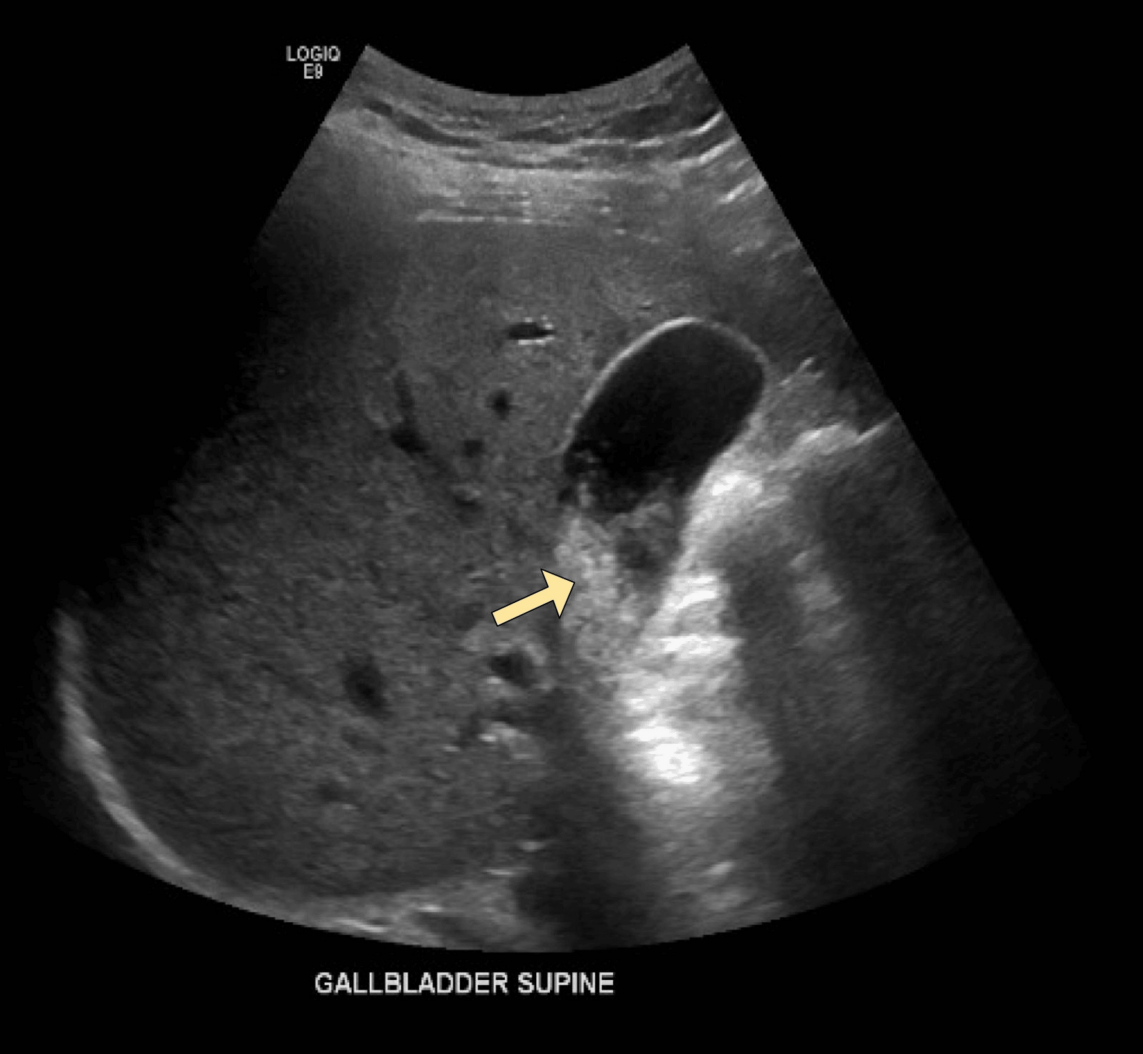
Abdominal US performed at initial presentation showing cholelithiasis (arrow) without evidence of acute cholecystitis US: ultrasound
